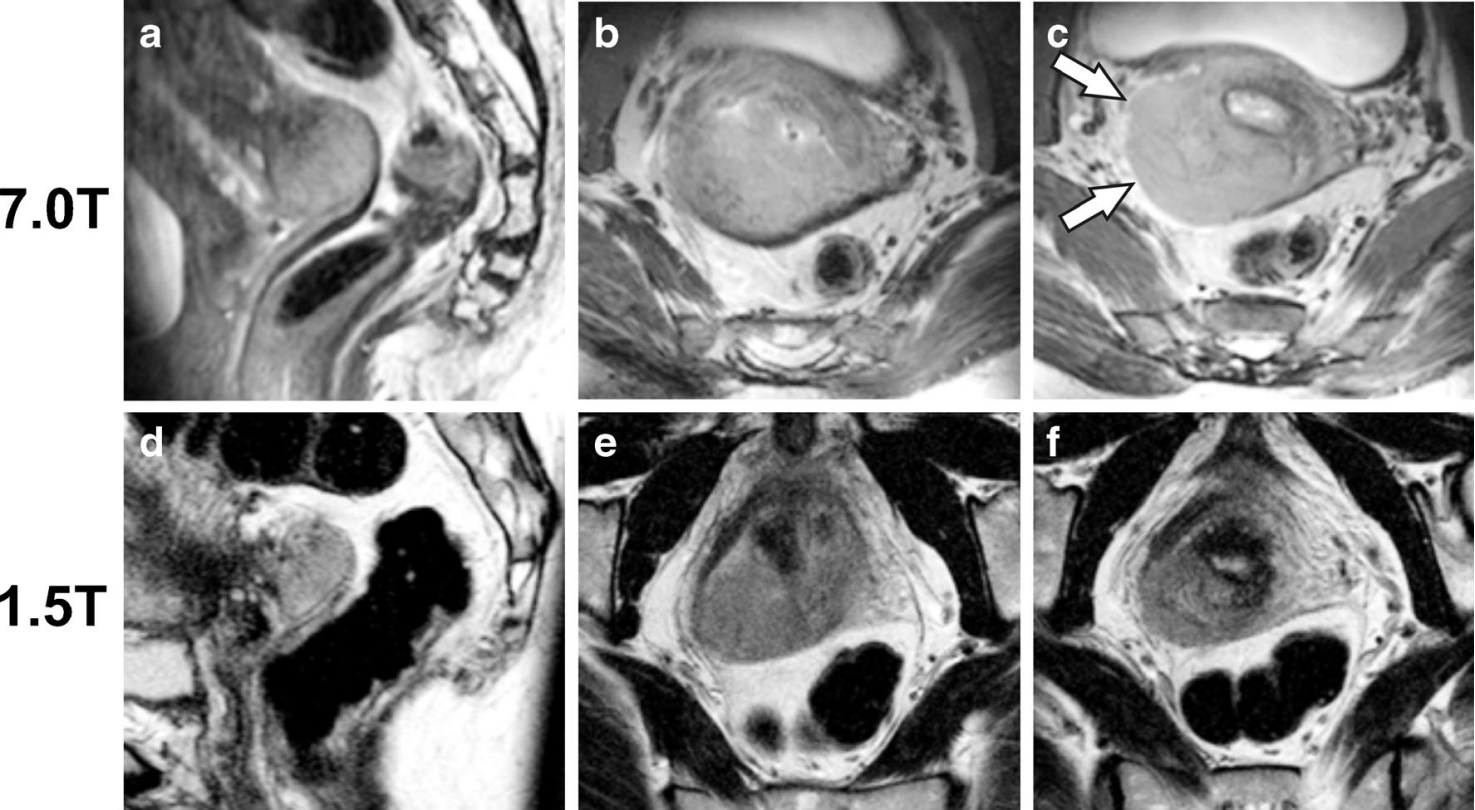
a Sagittal and b axial oblique T_2_-weighted acquisitions from the 7.0-T MRI of a 48-year-old woman diagnosed with an 80-mm poorly differentiated squamous cell carcinoma of the dorsal cervix. c. Slice from the same acquisition as b, though positioned 12 mm cranially. Parametrial invasion was judged absent at rectovaginal palpation, leading to a clinical stage IB2. However, the unclear tumour demarcation and absent T_2_ hypointense fibrostromal ring on the right (*arrows*) are suggestive of right-sided parametrial invasion (i.e. stage IIB). d-f The matched T_2_-weighted axial oblique slices from the clinical 1.5-T MRI, created 24 days earlier, are provided for comparison
